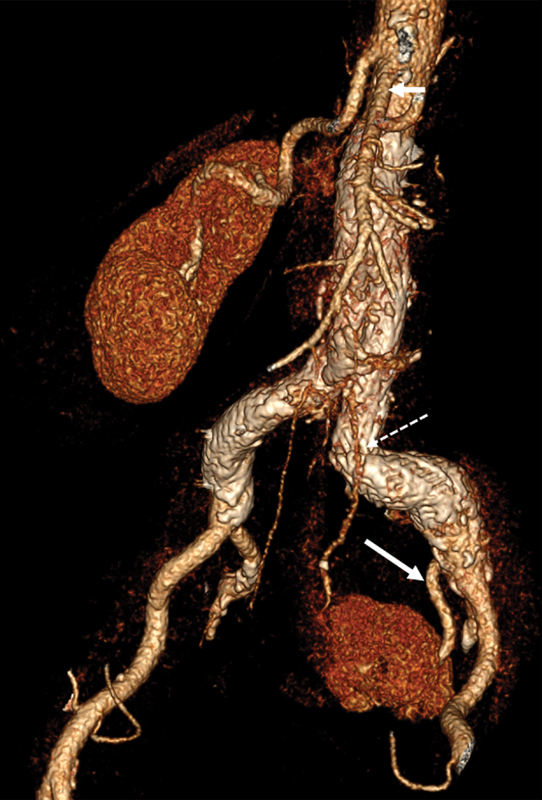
Three-month postoperative computed tomography angiography depicting the normal flow in the distal left renal artery (LRA; solid arrow). The proximal LRA is slightly opacified, probably via antegrade flow from the Type IIb endoleak (dotted arrow).
